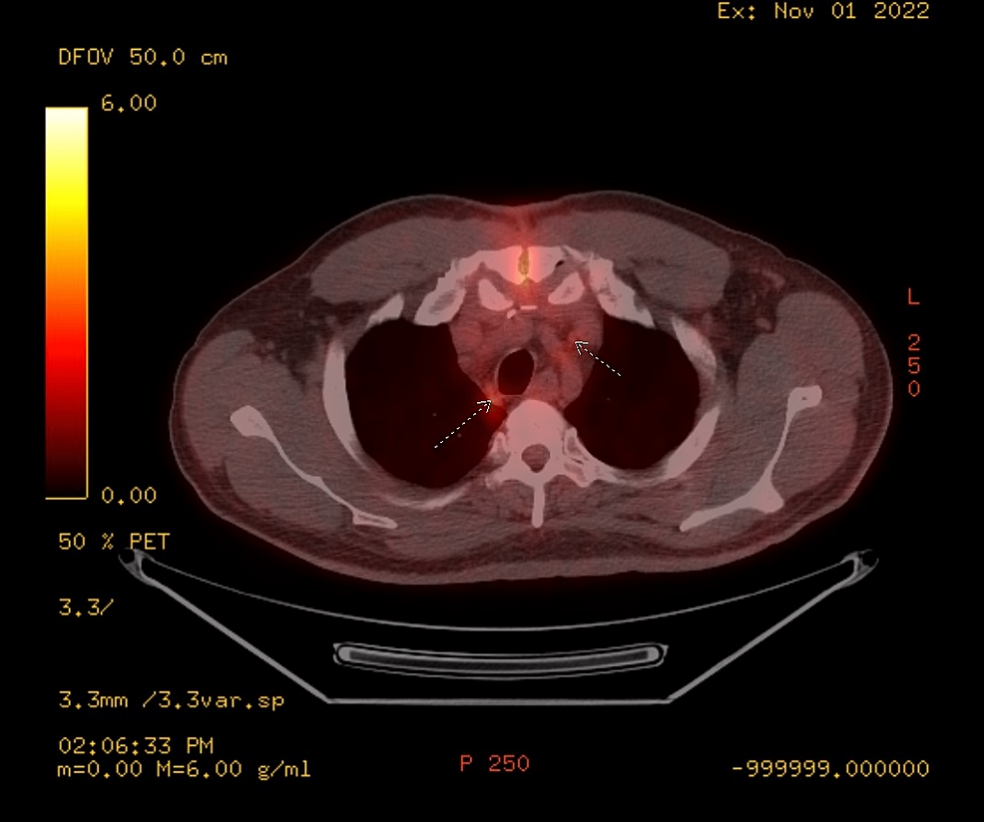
Post-chemotherapy PET/CT scan showing mildly fluorodeoxyglucose (FDG)-avid mediastinal lymph nodes
